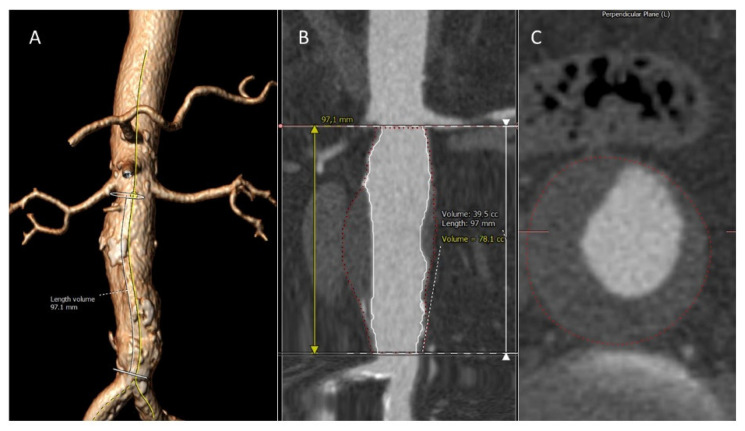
Volumetric image worksheet. (A) AAA 3D reconstruction with the centerline. (B) Results of the volumetry after the semi-automatic method. (C) CT slide showing the total volume area.
